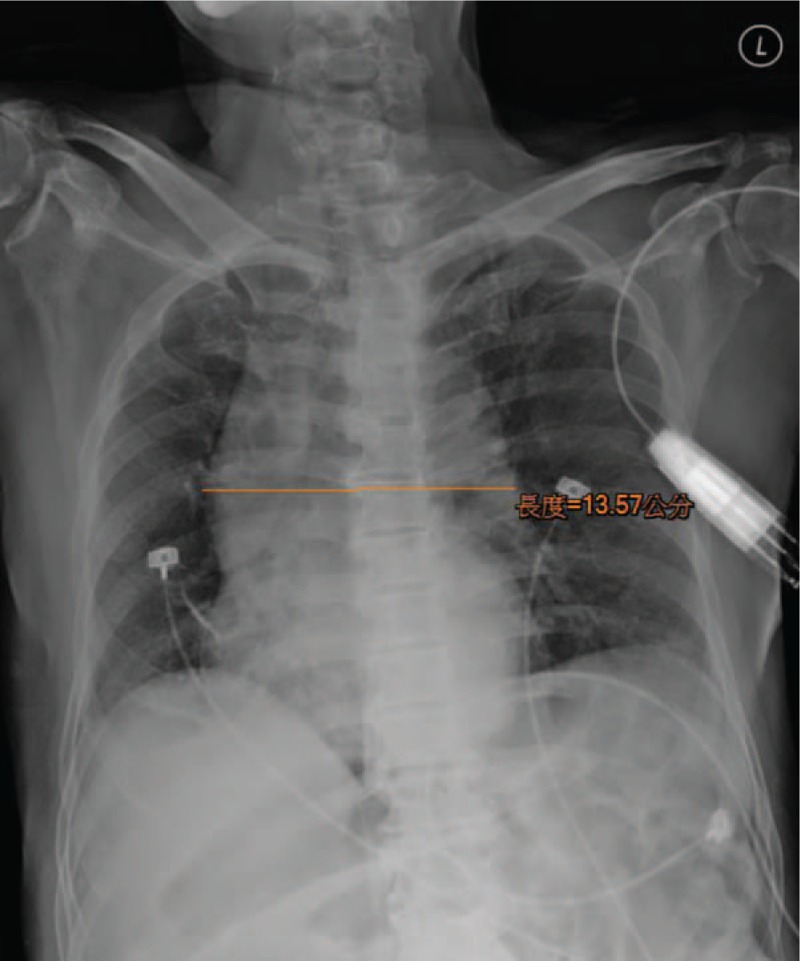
Chest radiograph showing mediastinal widening.
